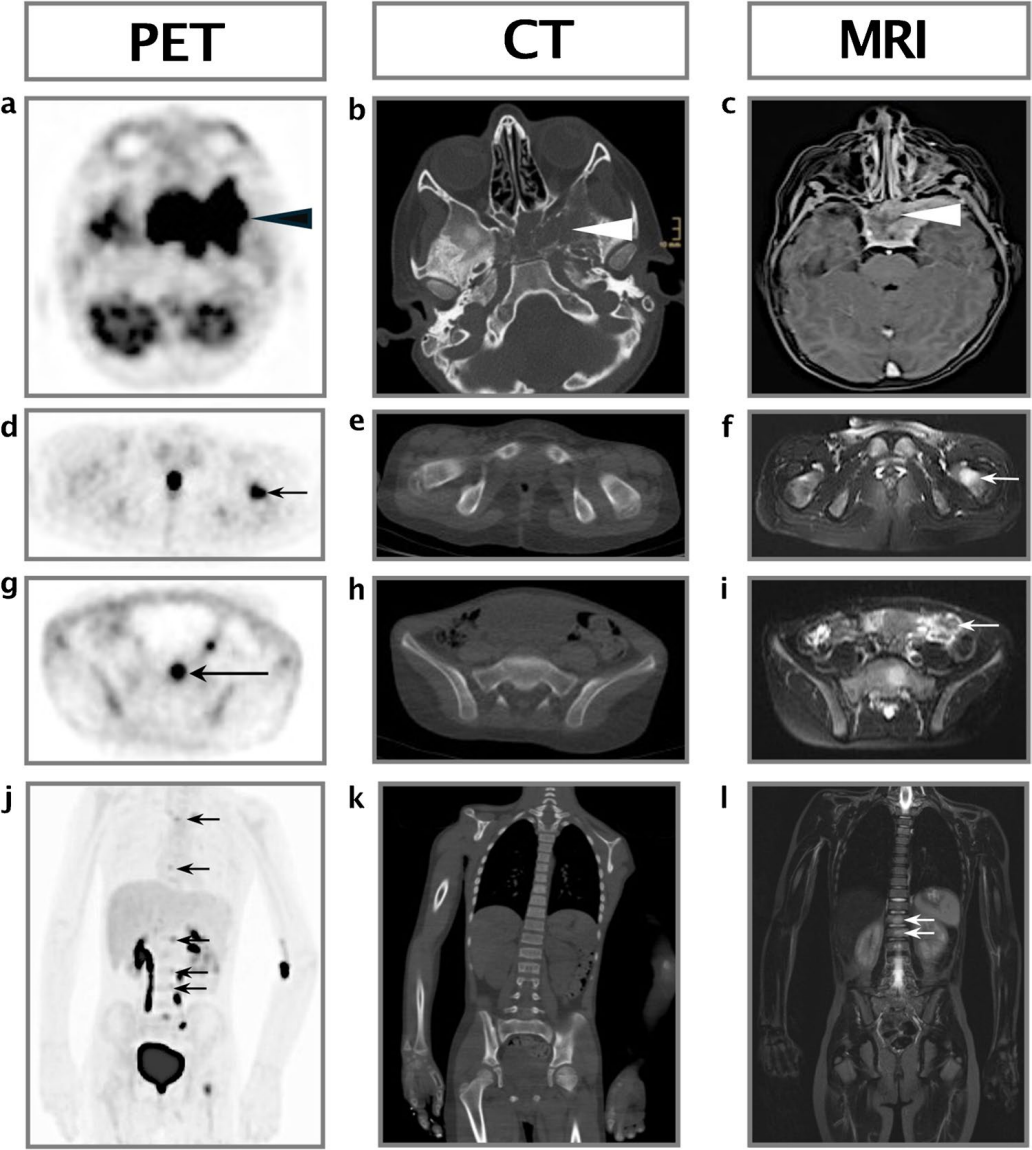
Findings in 2-[^18^F]fluoro-2-deoxy-D-glucose positron emission tomography (2-[^18^F]FDG-PET), computed tomography (CT) and magnetic resonance imaging (MRI) at the time of diagnosis in a 6-year-old boy with metastatic rhabdomyosarcoma. a–c Axial plane 2-[^18^F]FDG PET image (a) shows the large primary tumor at the skull base with 2-[^18^F]FDG uptake above the mediastinal blood pool (*arrowhead*). Axial plane CT image viewed on bone windows (b) and axial plane T2-weighted contrast MRI image (c) show the primary tumor, which extends from the sella turcica into the fossa infratemporalis and is associated with a large soft tissue mass (*arrowheads*). d–f Axial plane 2-[^18^F]FDG PET image (d) shows a 2-[^18^F]FDG-avid lesion in the left femur (*arrow*). Axial plane CT image viewed on bone windows (e) and axial plane T2-weighted MRI image without contrast (f) do not reveal changes in trabecular bone structure or an associated soft tissue mass (*arrow*). g–i Axial plane 2-[^18^F]FDG PET image (g) demonstrates a 2-[^18^F]FDG-avid lesion in the sacrum (*arrow*). Axial plane CT image viewed on bone windows (h) and axial plane T2-weighted MRI image without contrast (i) do not reveal changes in trabecular bone structure or an associated soft tissue mass (*arrow*). j–l Coronal plane 2-[^18^F]FDG PET image (j) shows 2-[^18^F]FDG-avid foci at thoracic (T) and lumbar (L) vertebrae (T4, T8, L1, L2, and L3). Coronal plane CT image viewed on bone windows (k) and T2-weighted MRI without contrast (l) do not show changes in trabecular bone structure or associated soft tissue masses (*arrows*)
